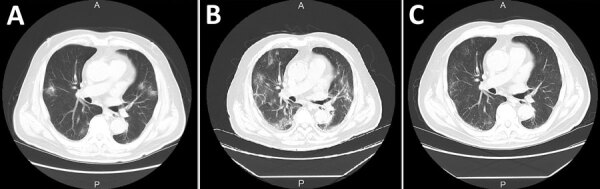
Chest computed tomography (CT) scan of a patient on hemodialysis diagnosed with positive reverse transcription PCR for severe acute respiratory syndrome coronavirus 2 in hemodialysis effluent, Japan. A) Chest CT at day 1 of hospitalization showing bilateral patchy ground glass opacities (GGO). B) Chest CT from day 5 of hospitalization showing worsening coronavirus disease 2019 (COVID-19) pneumonia with GGO expansion. C) Chest CT on hospitalization day 16 showing improvement of COVID-19 pneumonia; the patient was discharged on this day. A, anterior; P, posterior.
